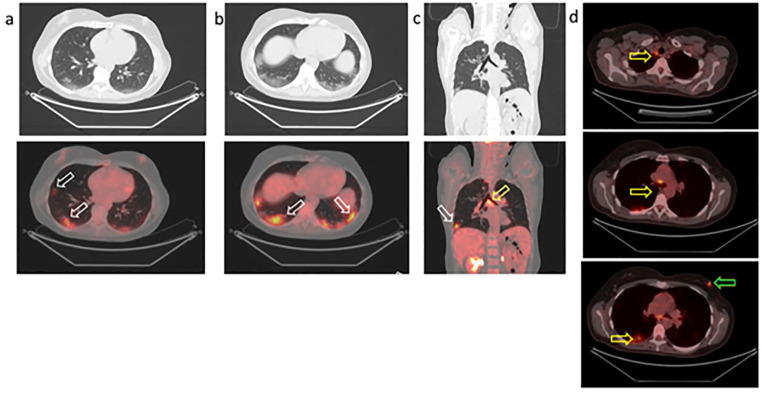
Positron emission tomography/computed tomography with ^18^F-FDG to
